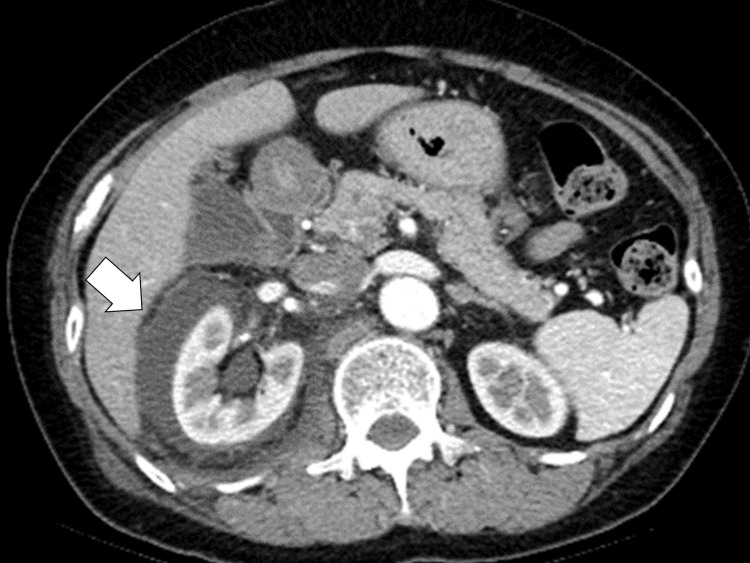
Contrast-enhanced abdominal computed tomography showing leakage of contrast medium during the excretory phase around the right kidney (white arrow)
